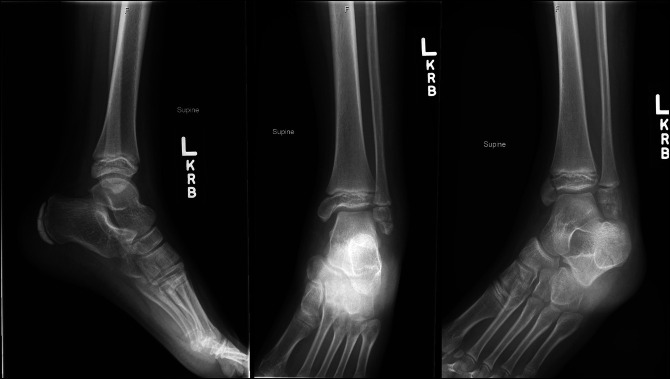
AP, mortise, and lateral view ankle radiographs of Case 1 after their injury demonstrating a displaced Salter-Harris type III medial distal tibia fracture and Ogden Type VII distal fibula fracture.
